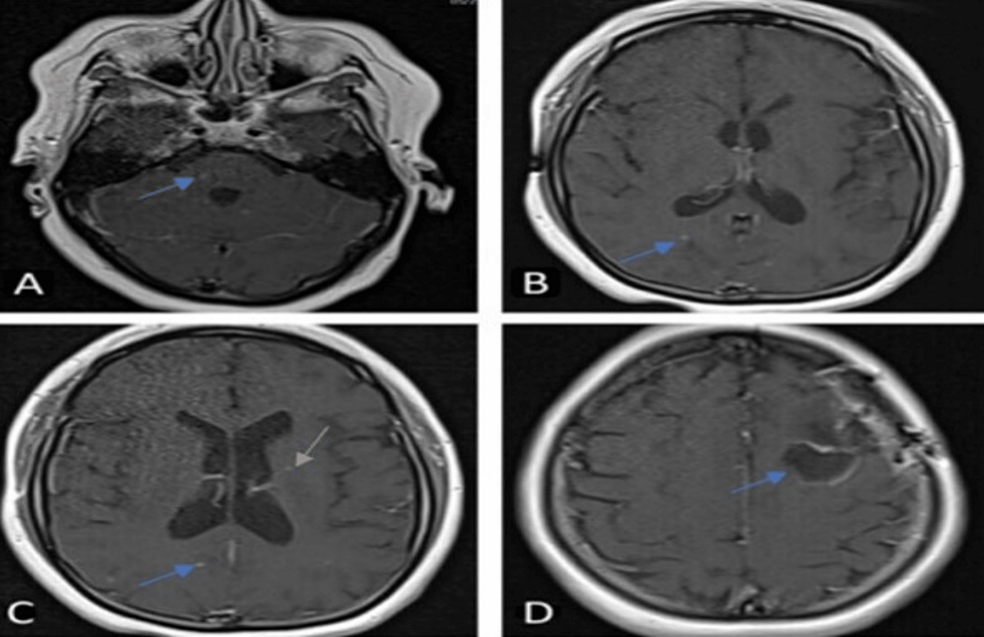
Brain MRI with contrast five weeks after discharge T1 with contrast axial sequence showing resolution of most lesion appreciated in Figure 1. (A) A small round enhancing lesion in the right medial pons (Blue arrow). (B) A small round enhancing lesion in the right subcortical occipital area (Blue arrow). (C) A small round enhancing lesion in the right subcortical occipital area (Blue arrow) and left corona radiata (orange arrow). (D) Expected progression of hematoma in the left frontal lobe (blue arrow).
